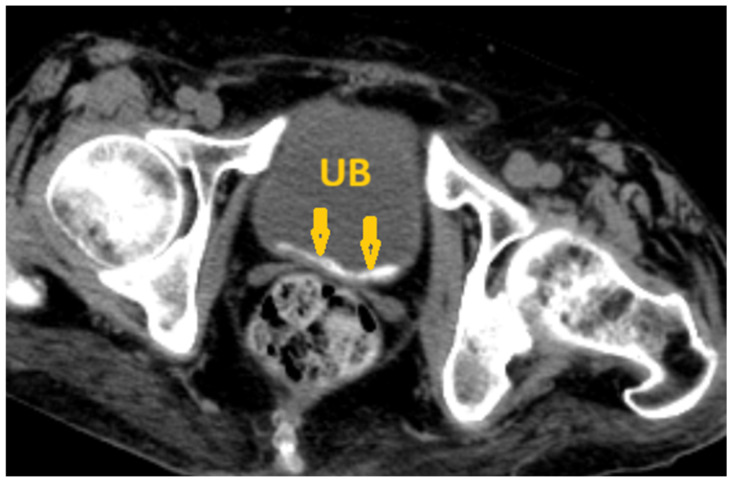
Selected non-contrast computed tomography axial image of a 40-year-old male patient shows linear calcification at the posterior wall of the urinary bladder (arrows), reflecting *Schistosoma* cystitis. UB: Urinary bladder.
